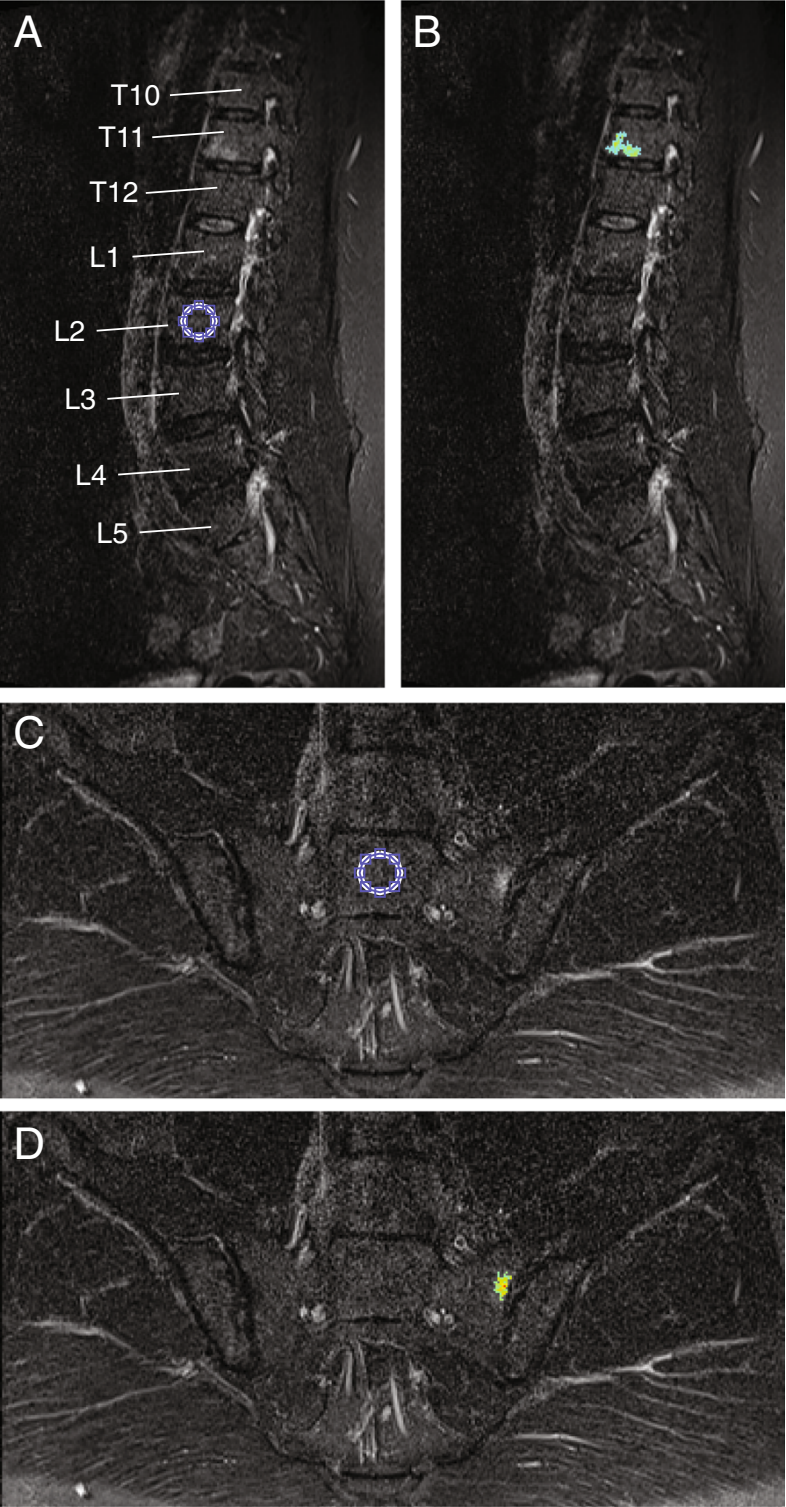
Example of placement of circular region of interest (ROI, ≥ 200 pixels) at the erector spinae muscles in short-tau inversion recovery (STIR) MR images of the spine (a) and gluteus maximus muscle in STIR MR images of the SI joints (c) of psoriatic arthritis patients for the normalization to signal from muscle tissue. For the selection of reference normal bone marrow signal as part of thresholding analysis, a circular ROI (≥ 200 pixels) was placed at a healthy vertebra in one slice of spinal images (a) and at the center of the first sacral vertebra in one slice in sacroiliac joint images (c). Example of thresholding of the volume of short-tau inversion recovery (STIR) hyper-intensity in a STIR MR image of the spine (b) corresponding to (a), and of sacroiliac joints (d) corresponding to (c). Vertebrae T10-L5 can be seen in (a) and (b). Inflammation was detected in T12. Iliac bones and sacrum are visible in (c) and (d)
